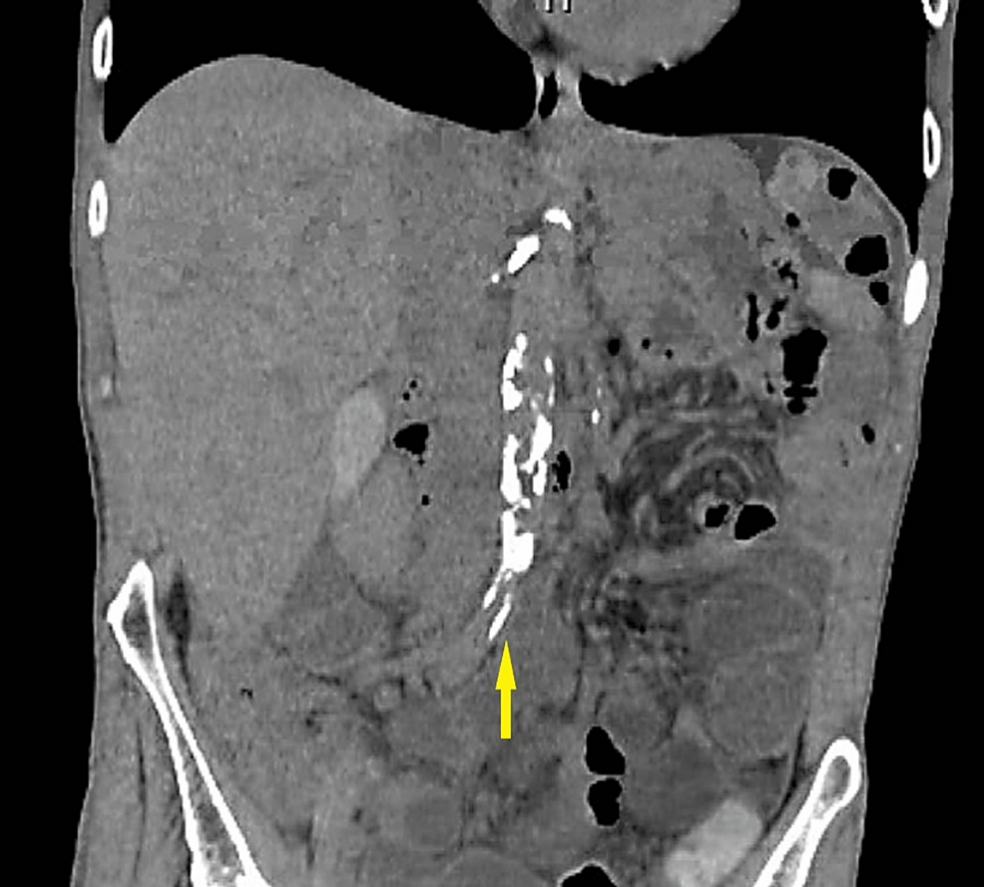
Severe atherosclerotic plaque burden of the aorta found on CT angiography (demarked by following the yellow arrow superiorly)
